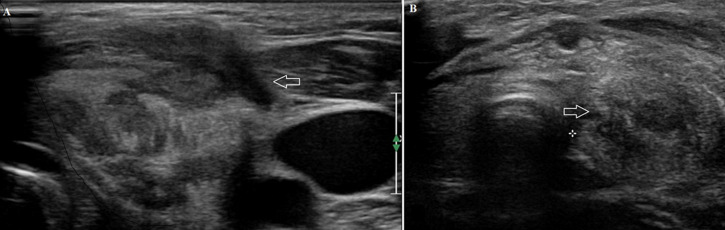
(A, B) first ultrasound imaging assessment, normal thyroid left lobe parenchyma echo texture is replaced from hypoechoic inflammatory lesion, through the findings is noticed a more hypoechoic elongated lesion which corresponds to the sinus track containing air bubbles that cause acousting shadowing
